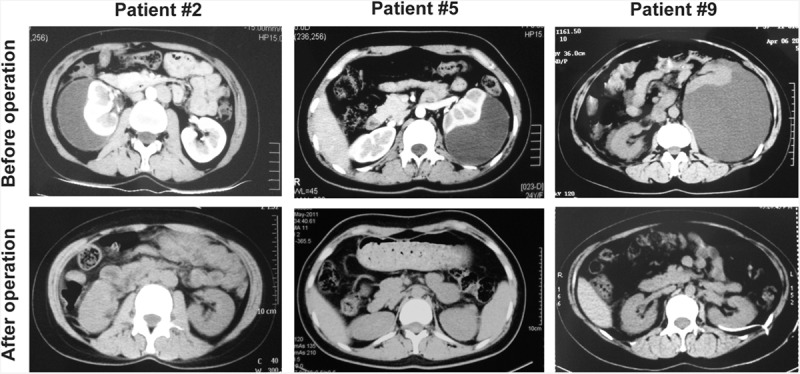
CT scan images before and after retroperitoneal laparoscopic renal capsulectomy for 3 individual patients with spontaneous renal subcapsular fluid collection. The contrast CT scan for patient #2 showed spontaneous renal subcapsular fluid collection with the maximum length of 9.0 cm in the right kidney, which largely regressed 1 month after the operation as demonstrated by CT scan. Patient #5 had spontaneous renal subcapsular fluid collection with a maximum length of 10.0 cm in the left kidney, 3 months after surgery, CT scans showed complete clinical regression. Patient #9 had spontaneous renal subcapsular fluid collection in both kidneys with a notably larger diameter of 15.5 cm on the left side; 6 months after retroperitoneal laparoscopic left renal capsulectomy, fluid collection almost disappeared in the left kidney. CT = computed tomography.
